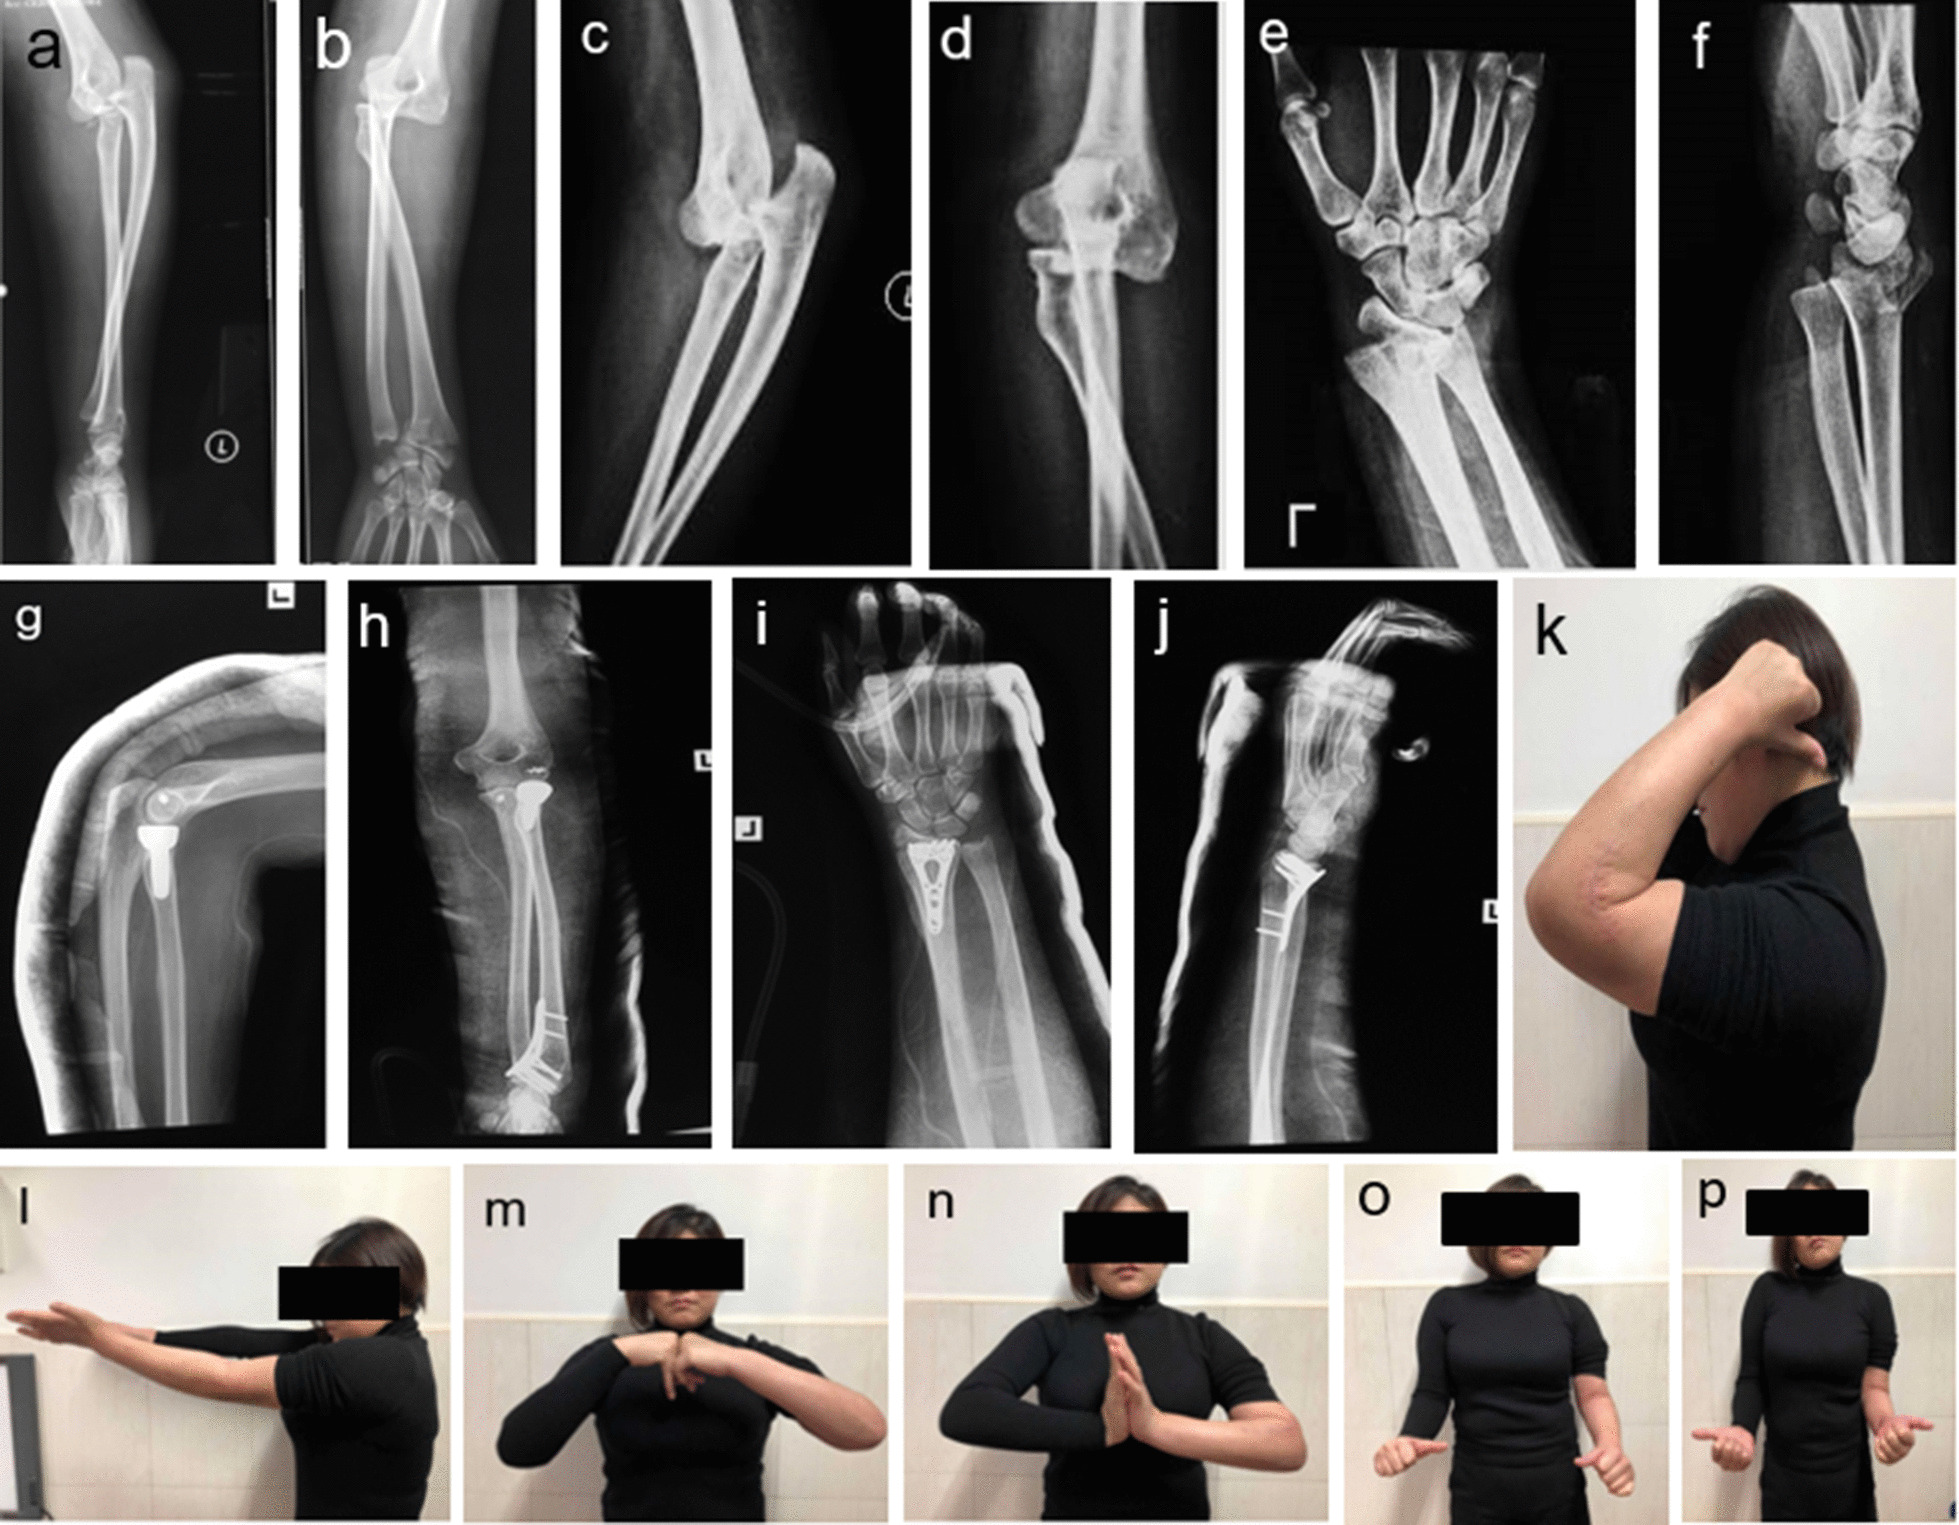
a–f: A 35-year-old female, whose preoperative radiographs demonstrated a distal radius and radius head fracture, fell from a two-story height. Furthermore, posterior dislocation of the proximal ulna with displaced radius head fracture was diagnosed as a sign of convergent elbow dislocation. g–j: With the failure of initial closed reduction under general anesthesia for elbow dislocation, open reduction was attempted to unlock the stuck radius head. ORIF of distal radius fracture followed after replacement of radius head and repair of lateral collateral ligament and joint capsule. k–p: At the latest follow-up after the injury, the patient had nearly full range of motion of her elbow and wrist
